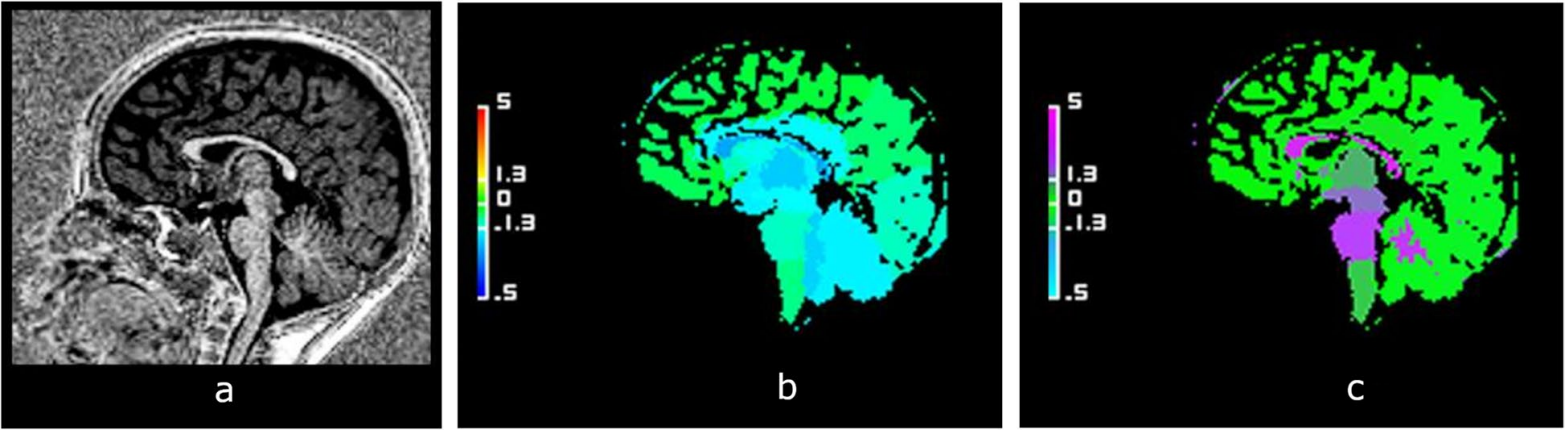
Example of hypotrophic corpus callosum (CC). Sagittal views of the brain midline in a boy aged 2 years and 11 months with neurodevelopmental delay. a High-contrast anatomical T1-weighted uniform image showing a hypoplasia of the corpus callosum. b Abnormal volumetric deviation map, with a CC appearing in light blue, *Z*-score = -2.86. c Increased T1 relaxometric deviation map, with a CC appearing in purple, *Z*-score = 3.20
